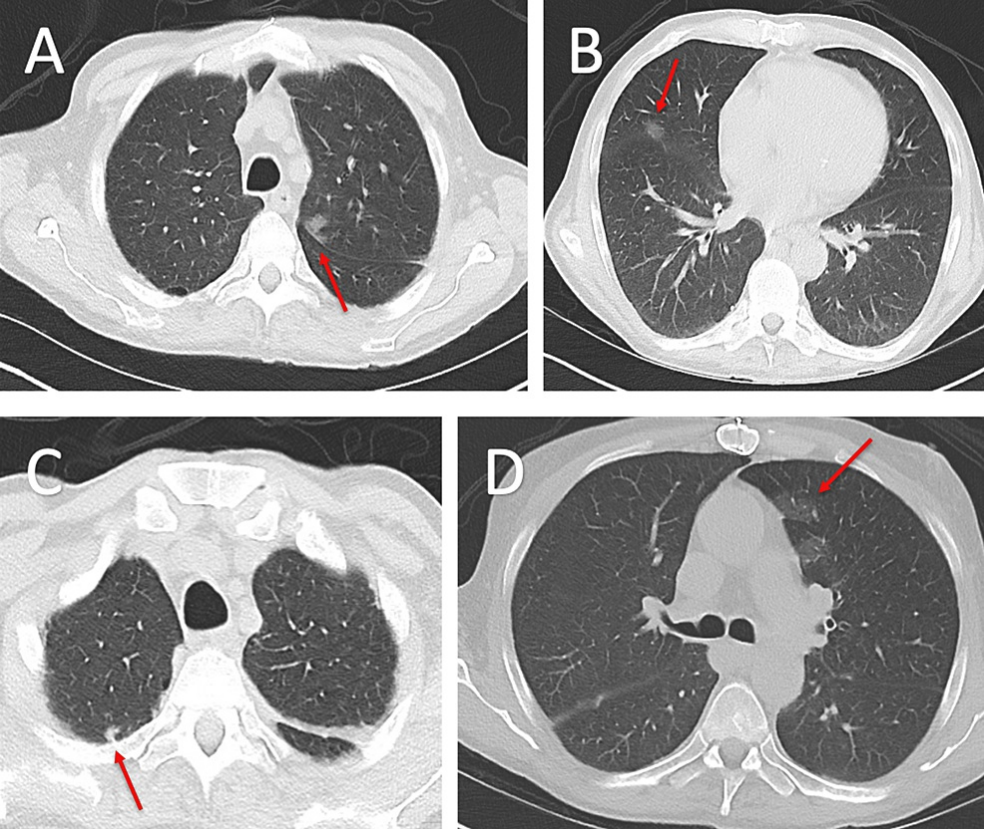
Axial chest CT demonstrates multiple ground glass opacities in bilateral lungs Multiple new-onset ground glass opacities (red arrows) are seen bilaterally in (A) the left upper lobe, (B) along the right horizontal fissure in the right middle lobe, (C) in the posterior right upper lobe associated with the pleura, and (D) in the lingula.
